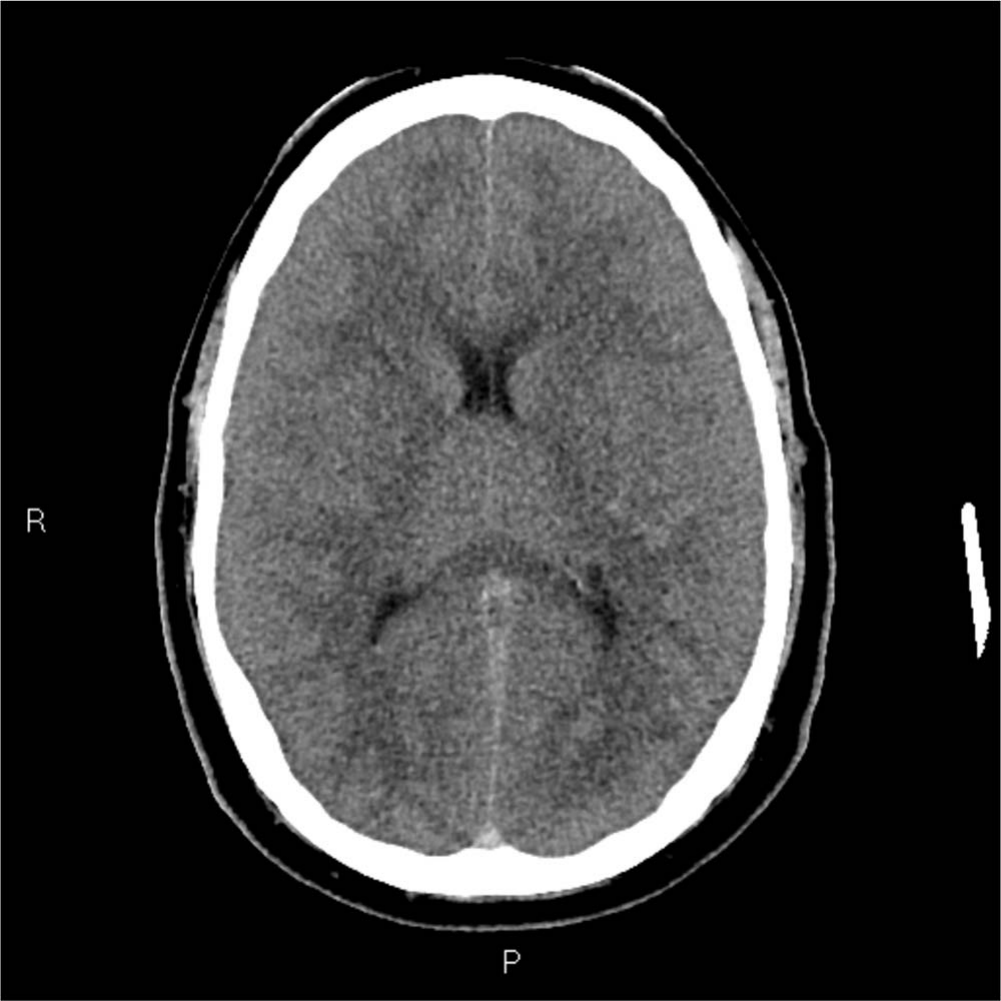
Axial non-contrast CT brain: Classical features of cerebral edema including effaced sulci and gyri, thin slit-like lateral ventricle with altered grey-white differentiation without a mid-line shift.
